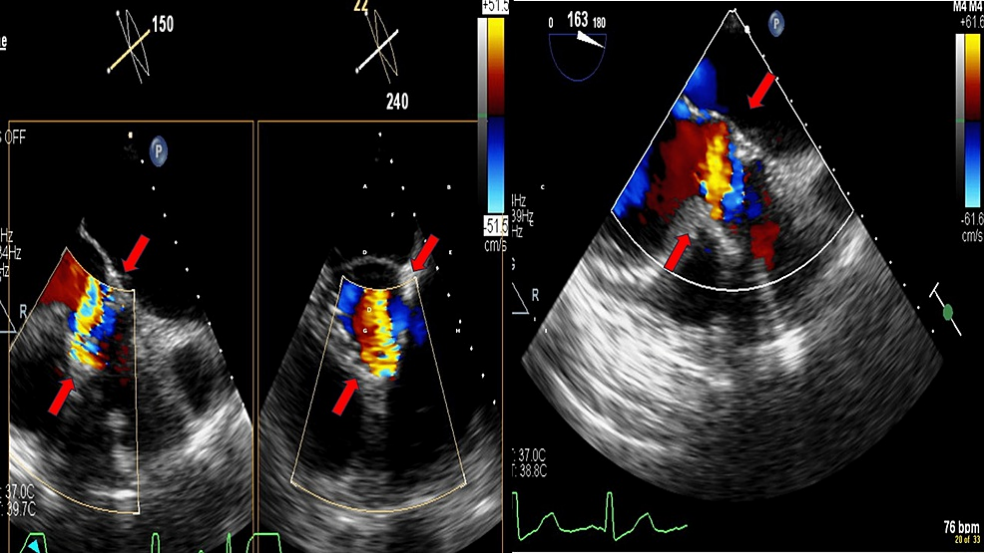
TEE demonstrating severe PVL, anterior to the bioprosthetic aortic valve ​(red arrows pointing) TEE, transesophageal echocardiogram; PVL, paravalvular leak
